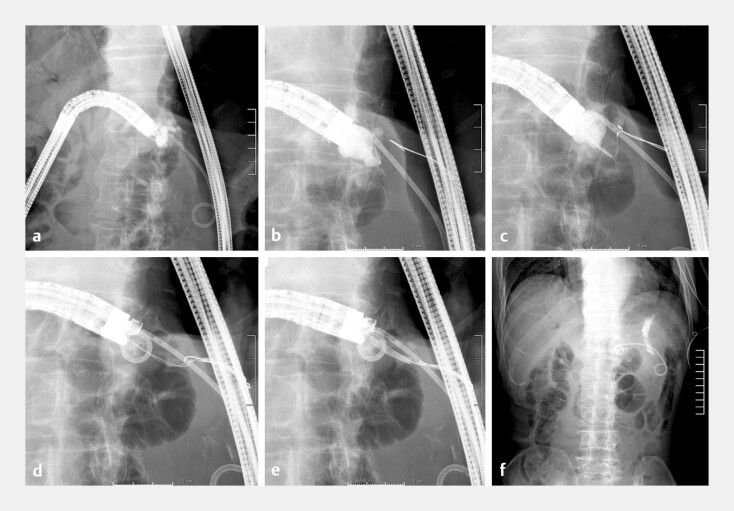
Endoscopic ultrasound-guided pancreaticojejunostomy.
